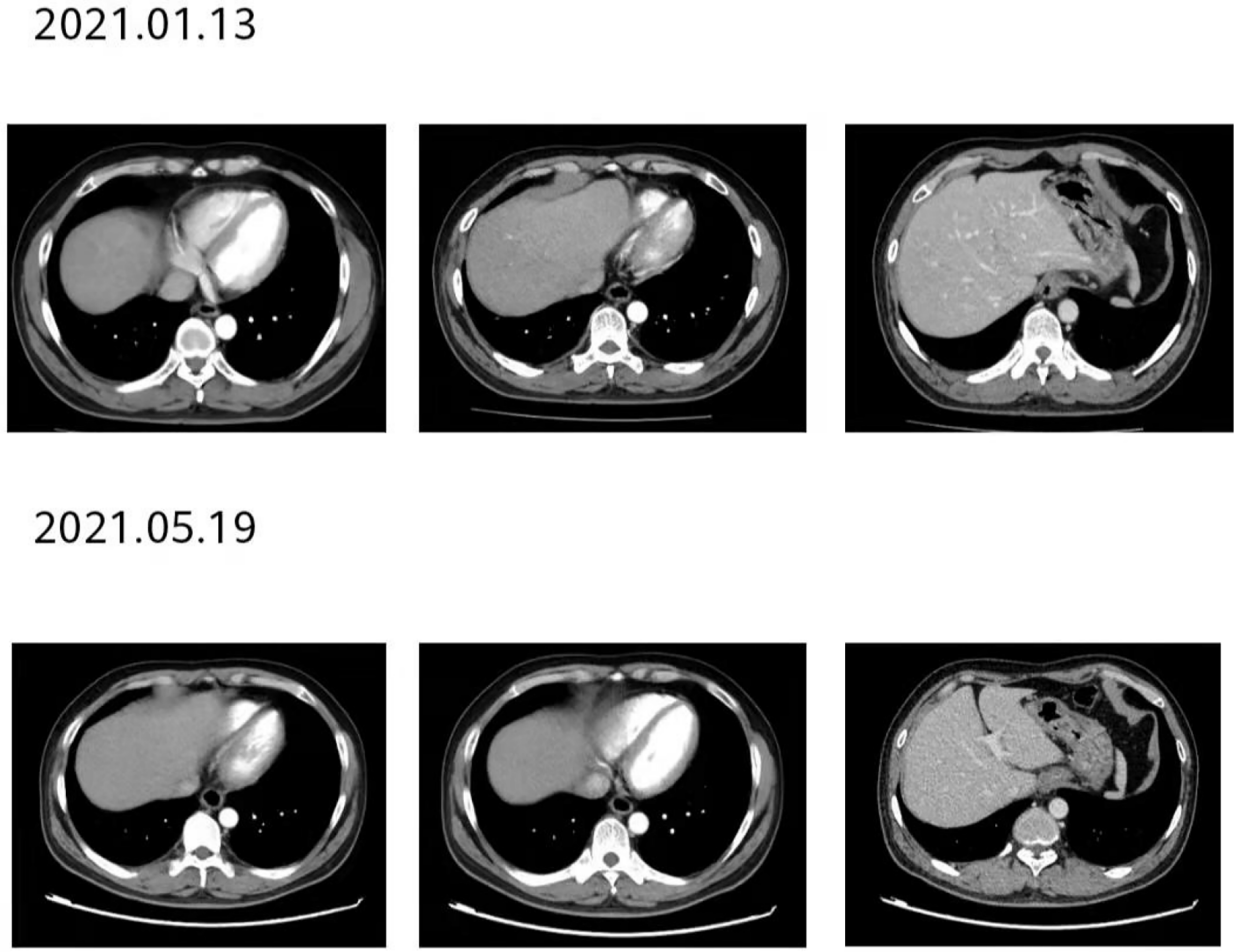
After 12 courses of treatment, re-examination of the second-stage enhanced CT of the lungs showed that the esophageal lesions were still smaller than before. Compared with that after 8 courses of treatment, the curative effect was evaluated as maintaining PR.
